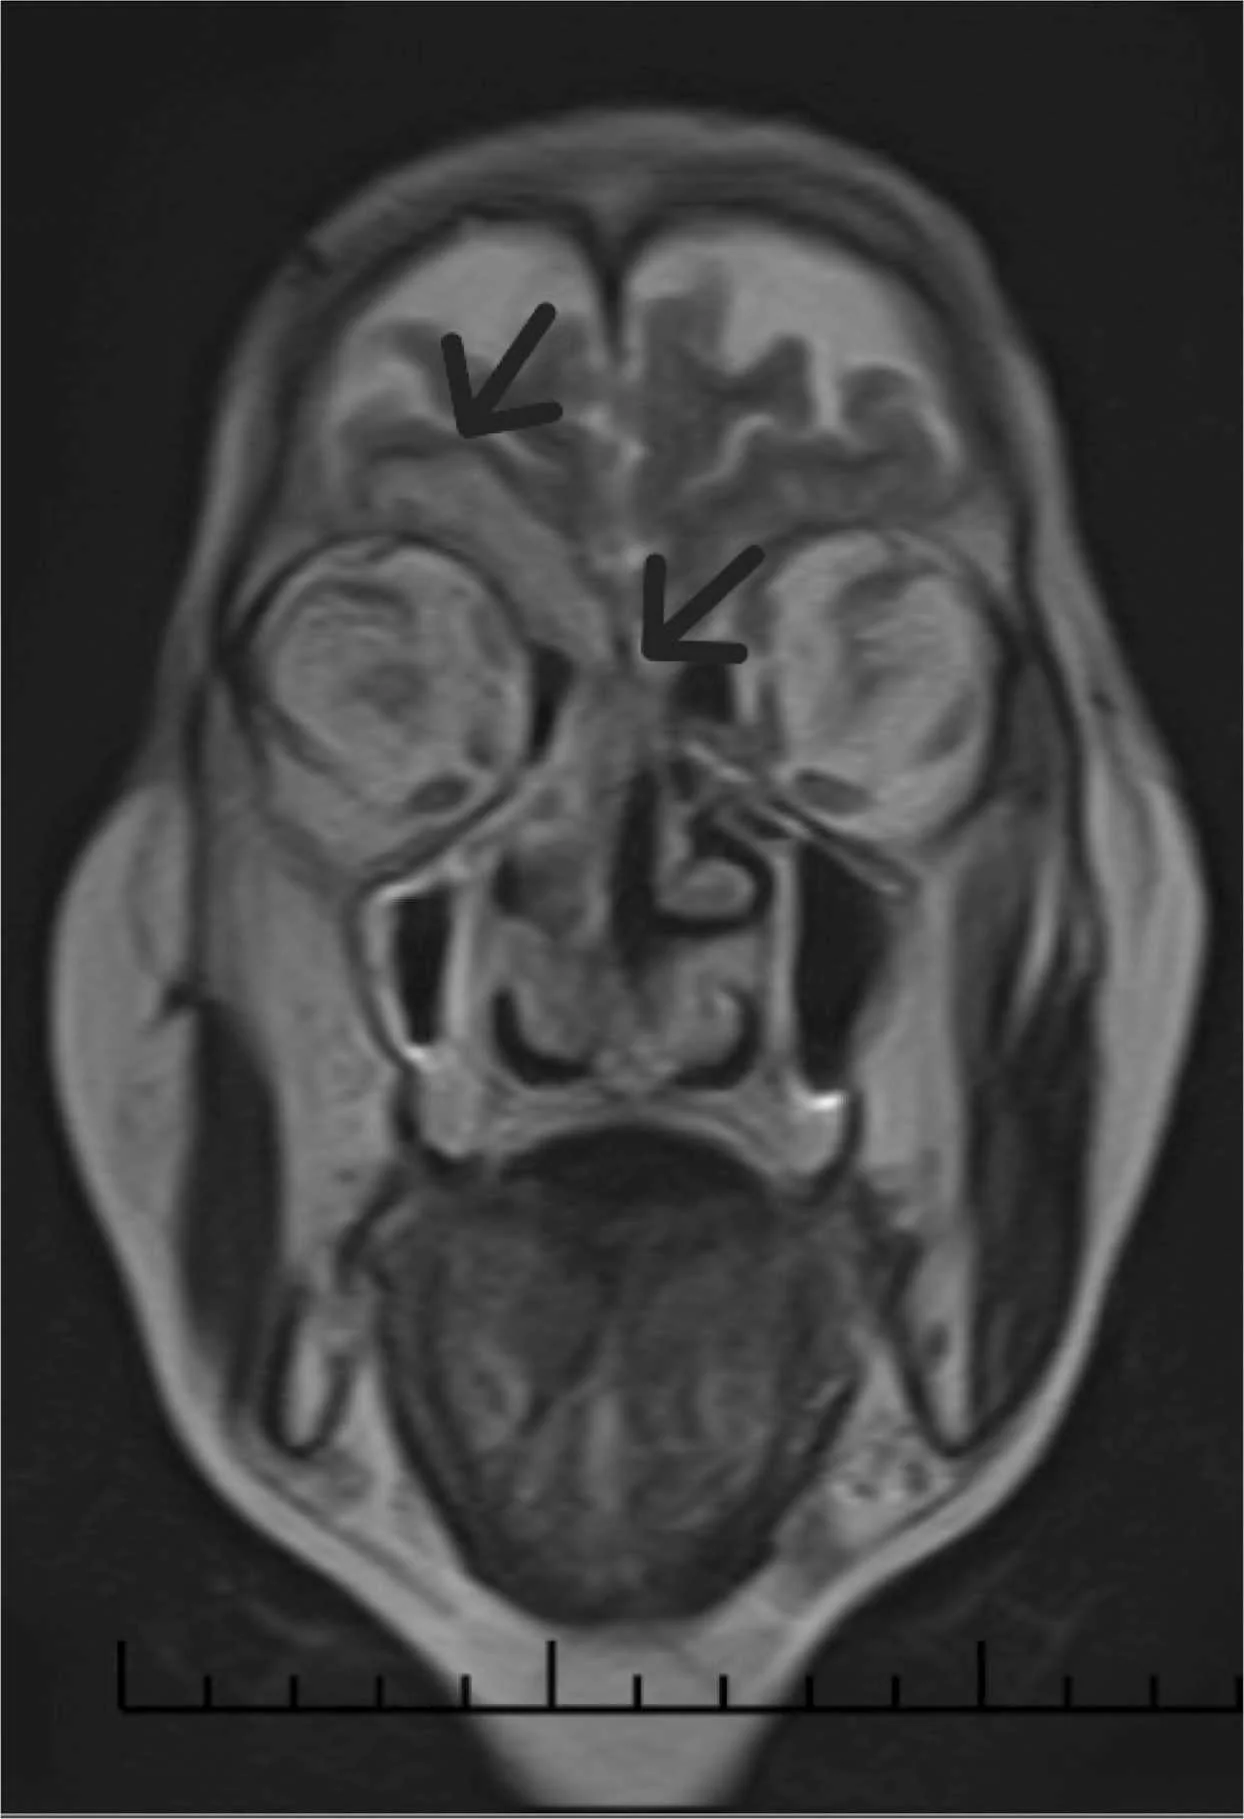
Orbital MRI demonstrating post-septal soft-tissue infiltration with intracranial extension into the right frontal lobe.
